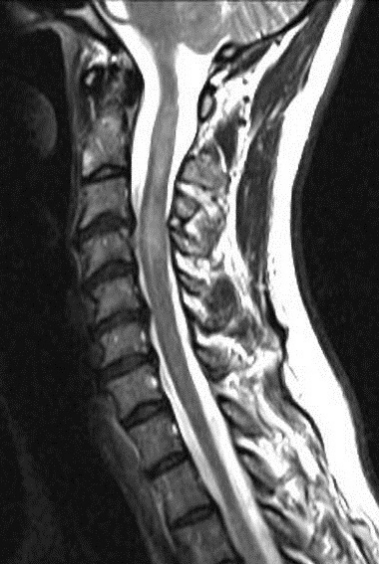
MRI spine showing hyperintensity in cervical cord C5 to C7 level (2)
